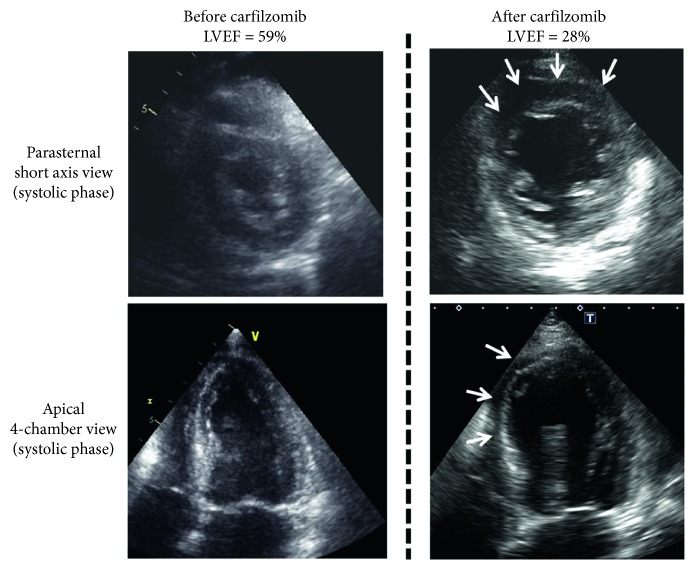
Transthoracic echocardiography beforeand after carfilzomib. After the administration of carfilzomib, the left ventricular ejection fraction decreased to 28% with diffuse wall motion abnormalities, particularly the anteroseptal segment.
